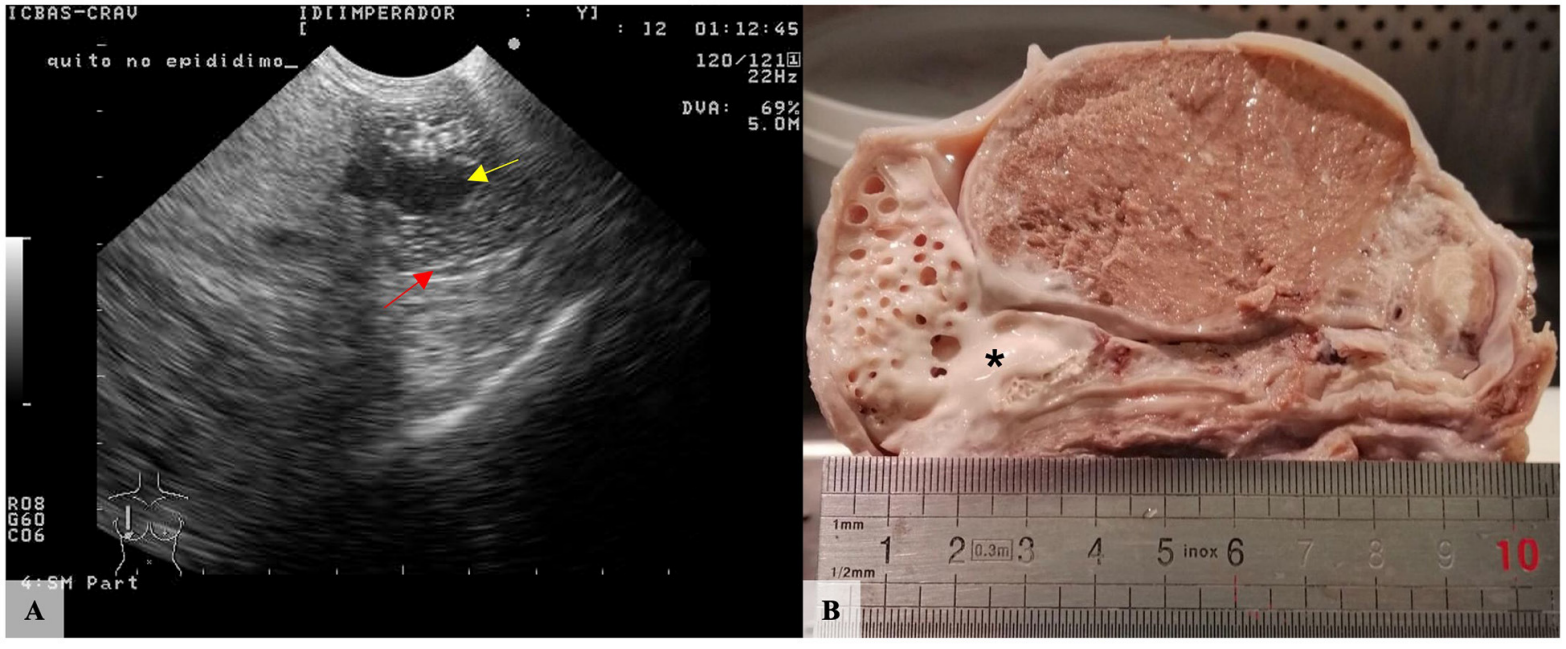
Purebred Lusitano stallion, right testis. (A) Ultrasound showed a well-circumscribed anechoic cystic structure (yellow arrow) in the epididymis. Red arrow: Epididymal wall. (B) Macroscopically, the largest cystic lesion (^*^) is filled with whitish and semen-like material located in the terminal portion of the body of the epididymis. Several smaller cavities were also identified in the tail of the epididymis.
